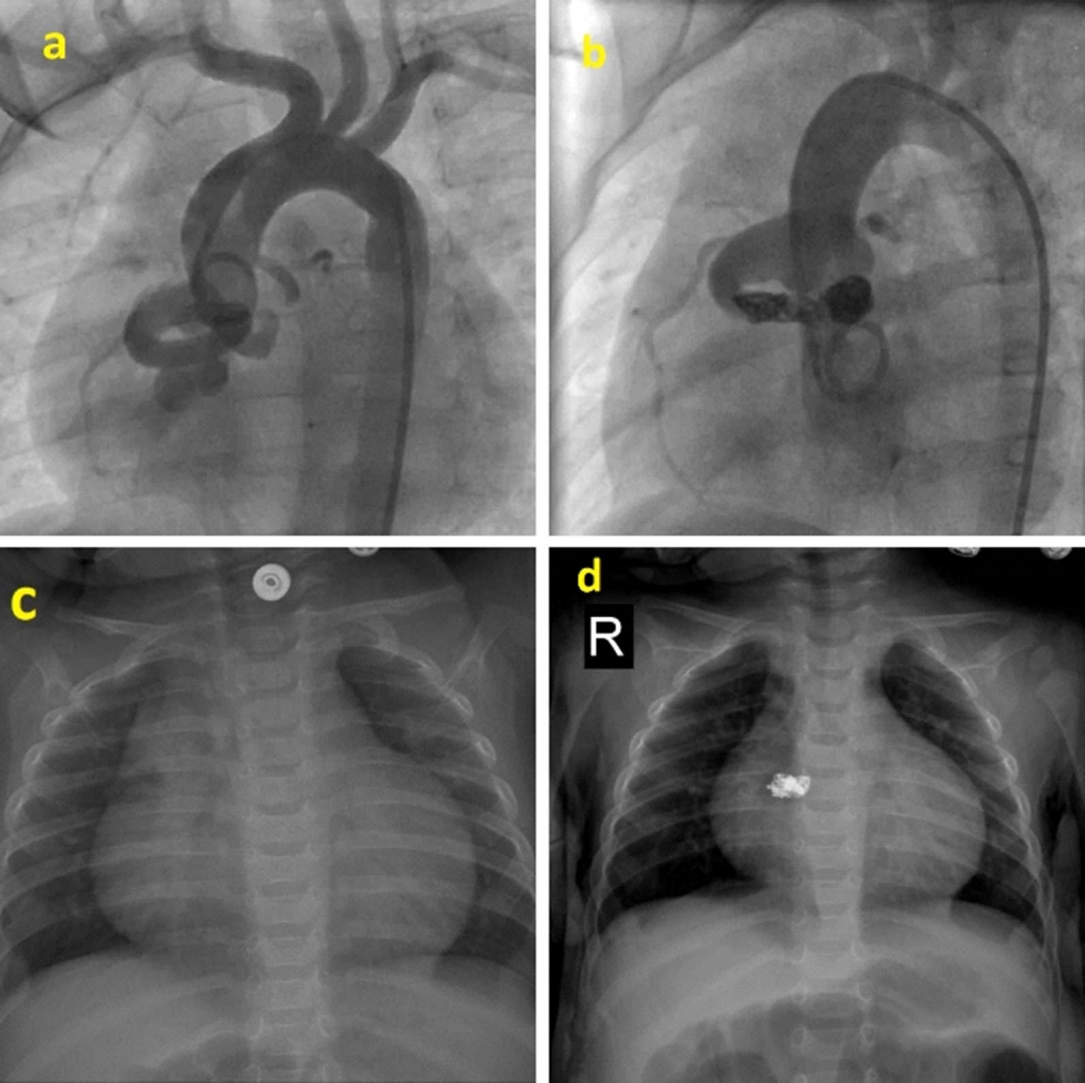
Fistula between RCA and RA which has been closed with 2 PFM coils via retrograde approach. a, b; Dilated fistula between RCA and RA, which has been closed with 2 pfm coils via retrograde approach. c, d; Reduced CT/ratio after the coil embolization
